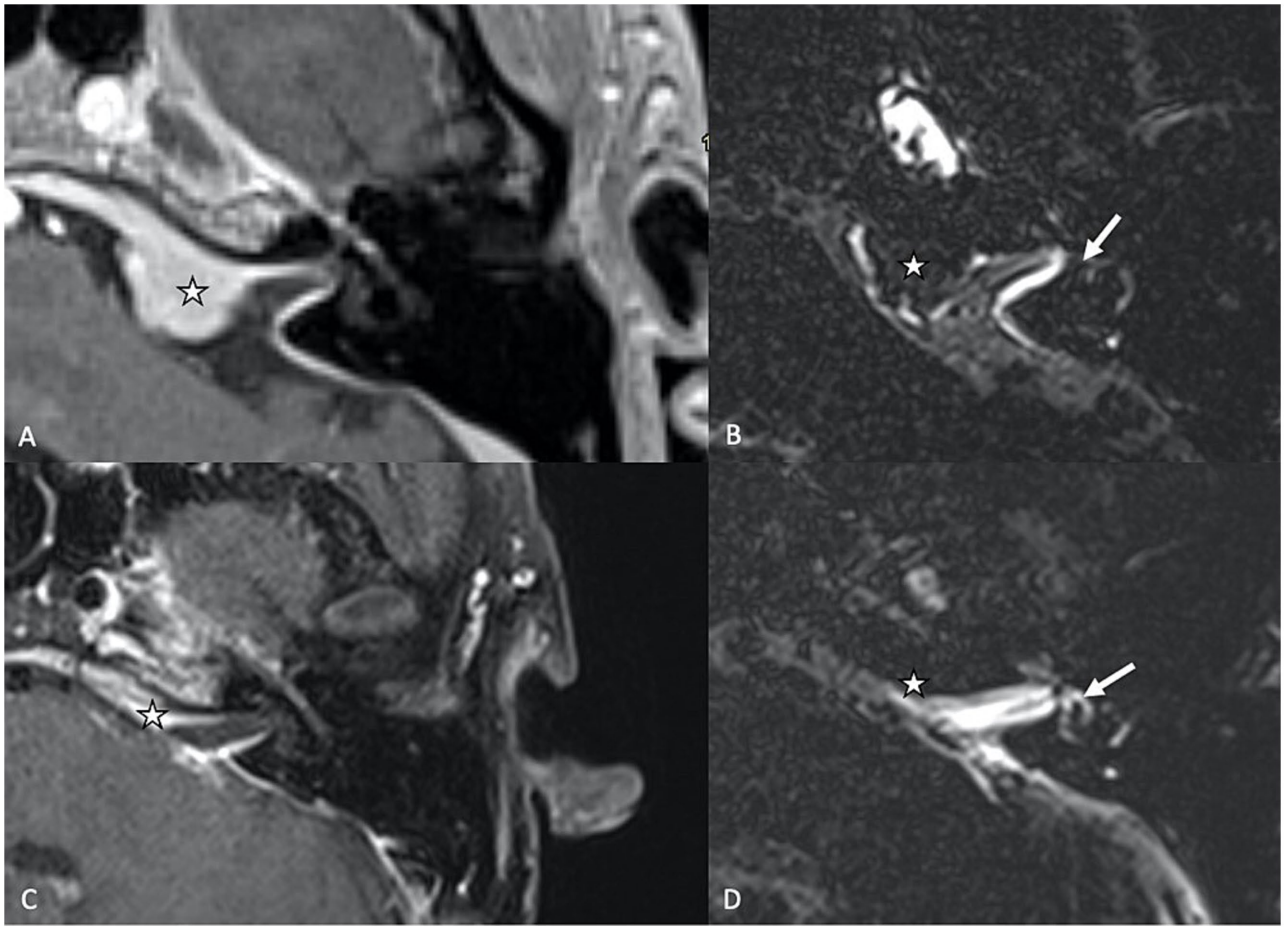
(A) Axial T1-weighed contrast-enhanced MR sequence showing a cerebello-pontine angle meningioma (star) extending into the internal auditory canal. (B) Delayed (4 h30) FLAIR image showing vestibular endolymphatic hydrops (grade 3) with full obliteration of the bony vestibule. (C) T1-weighed contrast-enhanced sequence showing residual scar tissue (star) tissue after surgical resection. (D) On the delayed FLAIR sequence, there is regression of the endolymphatic space dilation, which coincides with the clinical resolution of vertigo attacks for the patient.
